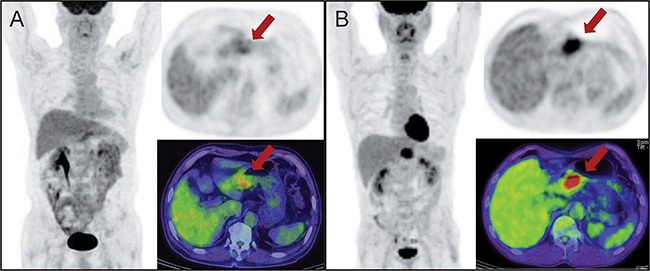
A. Preoperative 18F-PET/CT of a 64 year-old male shows mildly increased FDG uptake (SUV_max_ 3.6/TLR_max_ 2.0) in the gastric antrum. This patient was underwent subtotal gastrectomy and confirmed stage III gastric cancer (pT4aN3). The tumor was 6.0 × 5.5 cm sized, Bormann type II, poorly differentiated tubular adenocarcinoma. He followed up until 42 months without recurrence. B. A 55 year-old male, 18F-PET/CT for staging of gastric cancer presents localized intense hypermetabolic activity at the gastric antrum. The SUV_max_ and TLR_max_ of the tumor was 10.3 and 6.4, respectively. After subtotal gastrectomy, 4.5 × 3.0 cm sized, Bormann type III, poorly differentiated tubular adenocarcinoma was confirmed and the pathologic stage was pT4aN3. This patient had a recurrence 19 months after the surgery.
